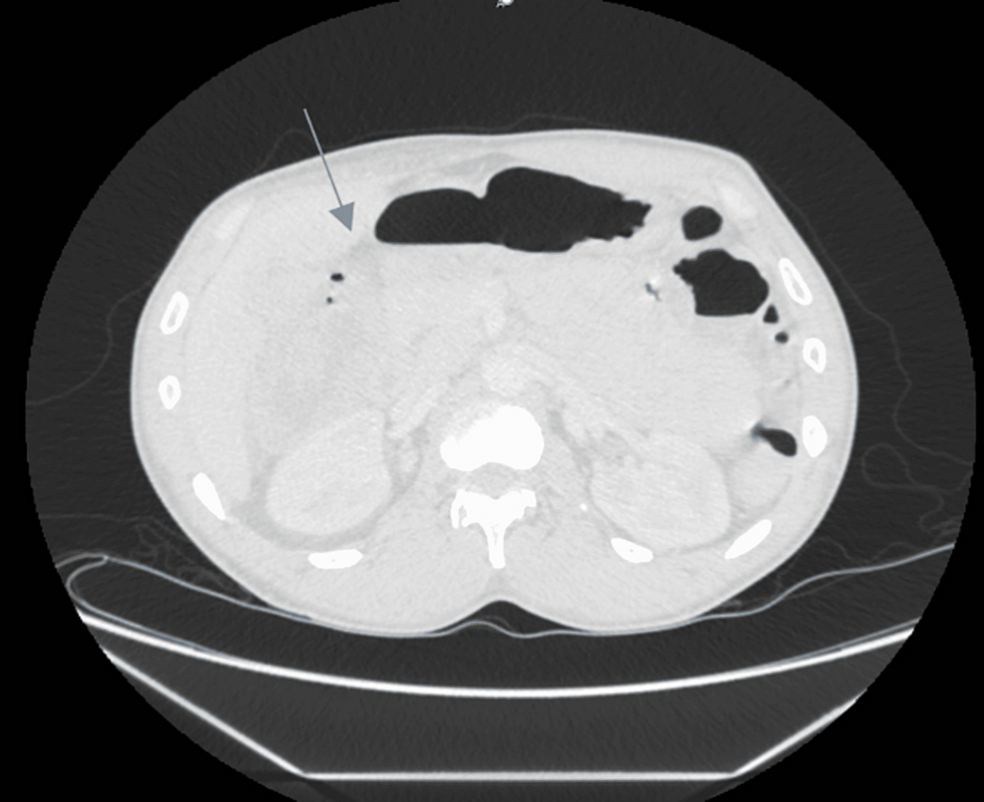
CT of the abdomen and pelvis Small bowel dilatation with the arrow pointing to the transition point
